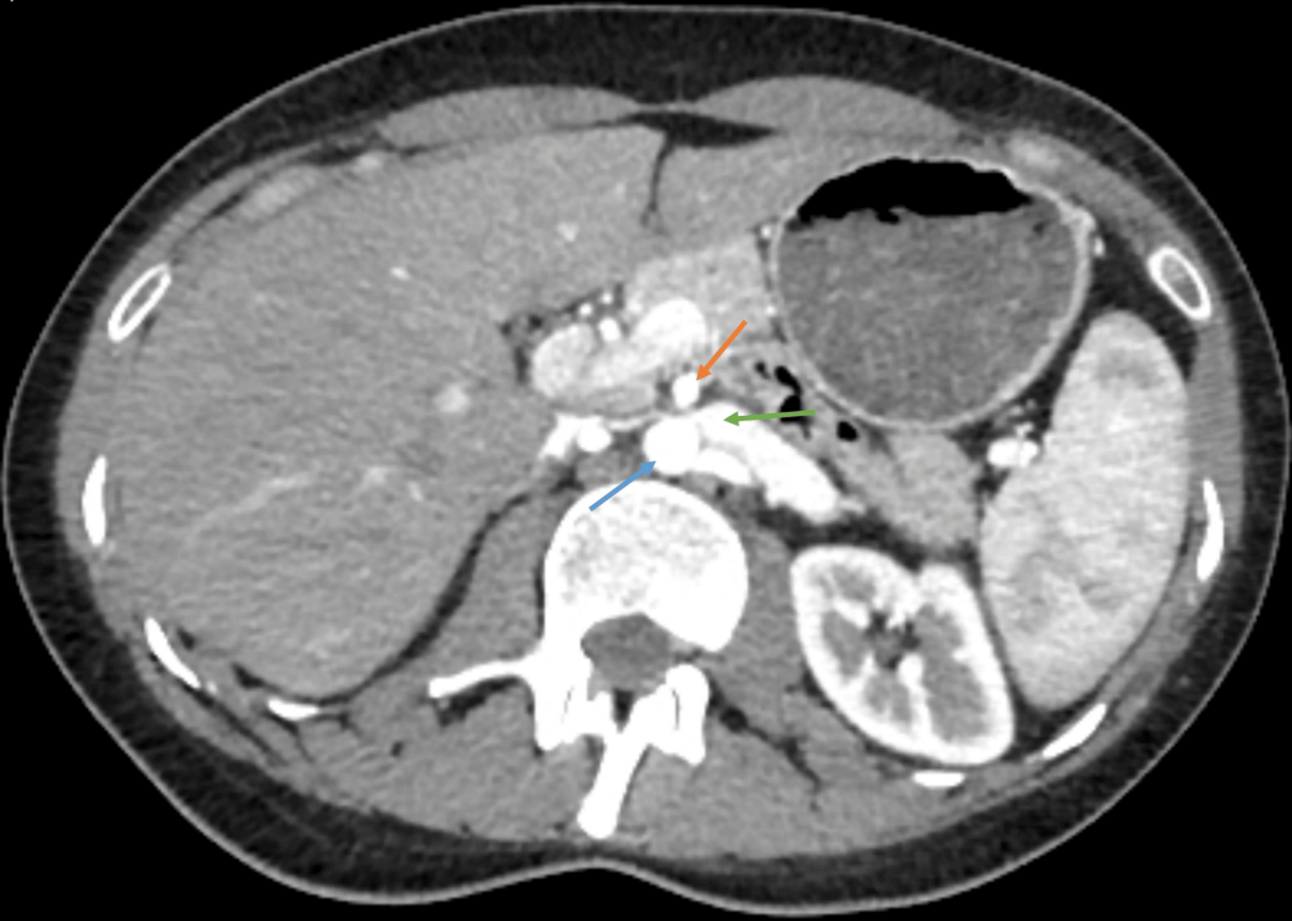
Axial CT image showing narrowing of the left renal vein (green arrow) as it crosses to the left between the SMA (orange arrow) and the aorta (blue arrow) CT: Computed Tomography; SMA: Superior Mesenteric Artery
